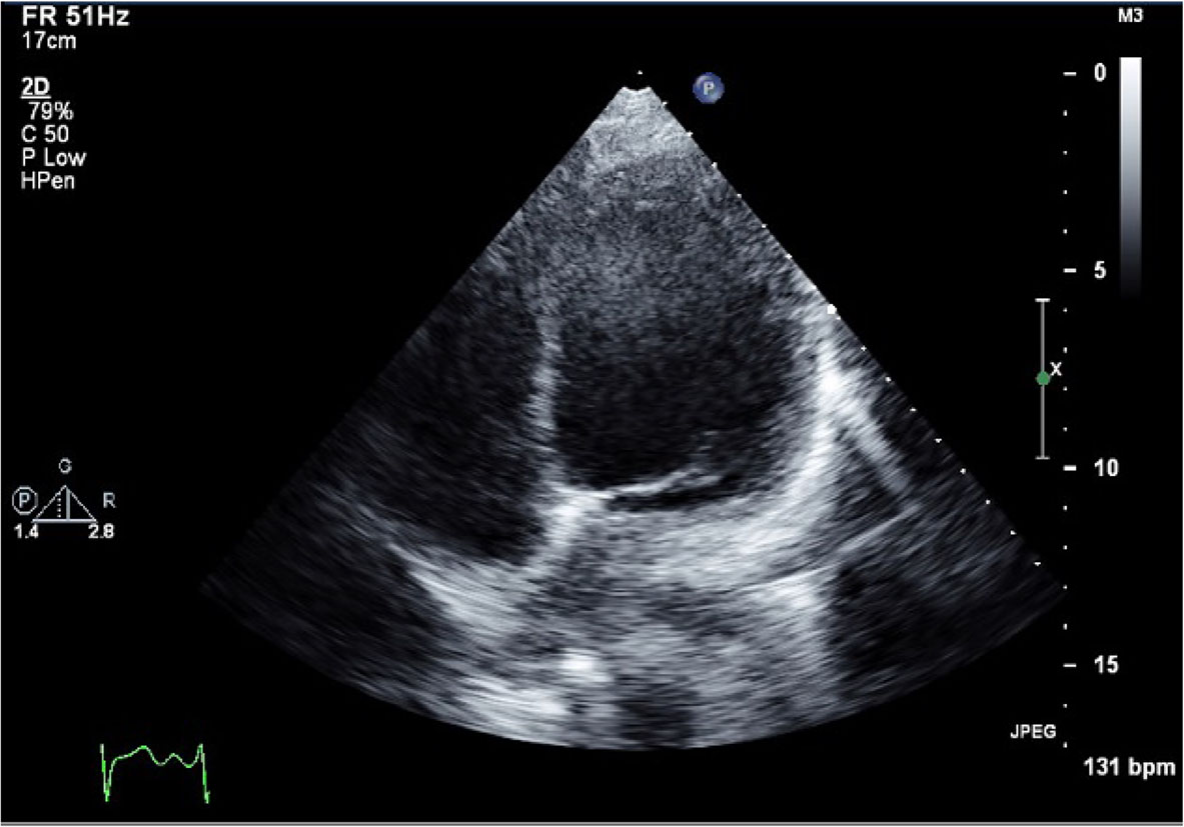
Transthoracic Echocardiography at admission demonstrates a dilated left ventricle dimension. Apical 4 Chambers view at the end of the diastole
